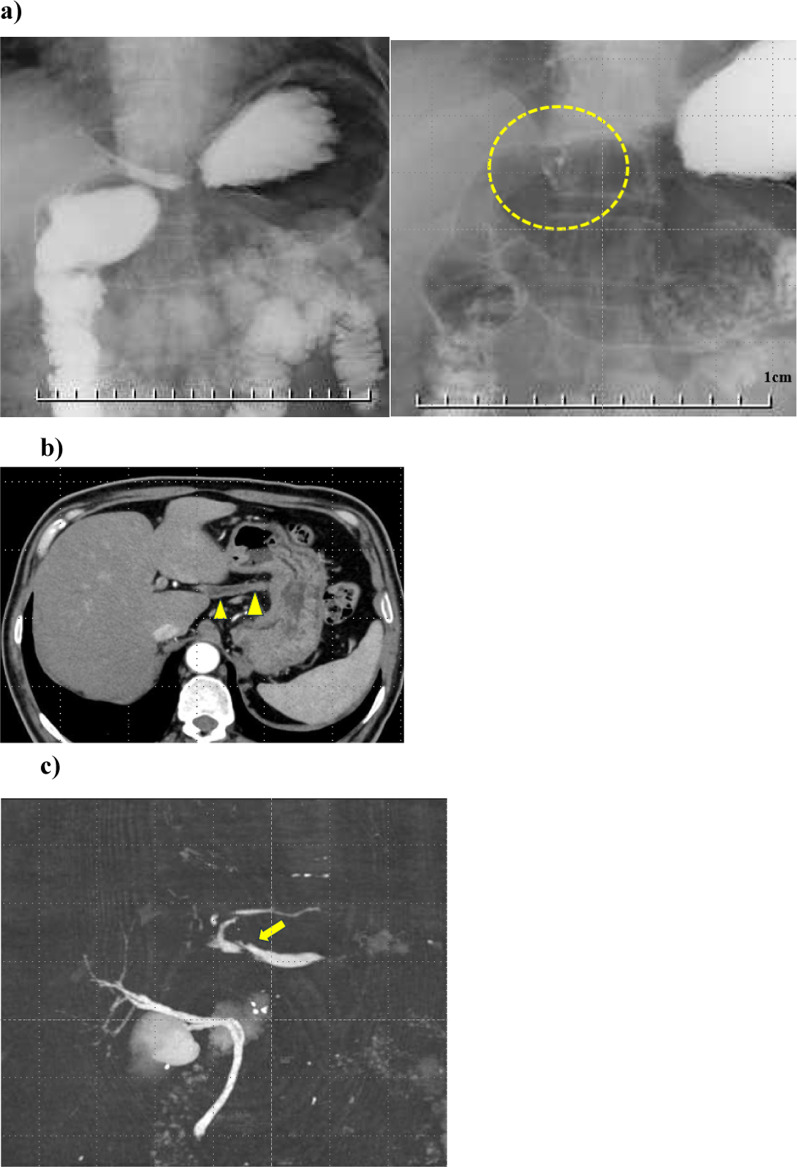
Pre-operative tests. a Upper gastrointestinal examination. b Computed tomography image showing ectopic bile drainage in the stomach. c Magnetic resonance cholangiopancreatography showing a signal defect in the ectopic bile duct and no communication between the left and right intrahepatic bile ducts
